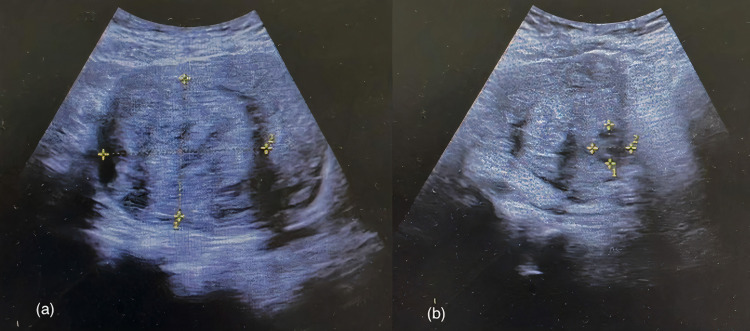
Transvaginal ultrasound showing intramural uterine fibroids (yellow markers) with sizes: (a) 5.63 x 6.70 cm (b) 1.68 x 1.68 cm
